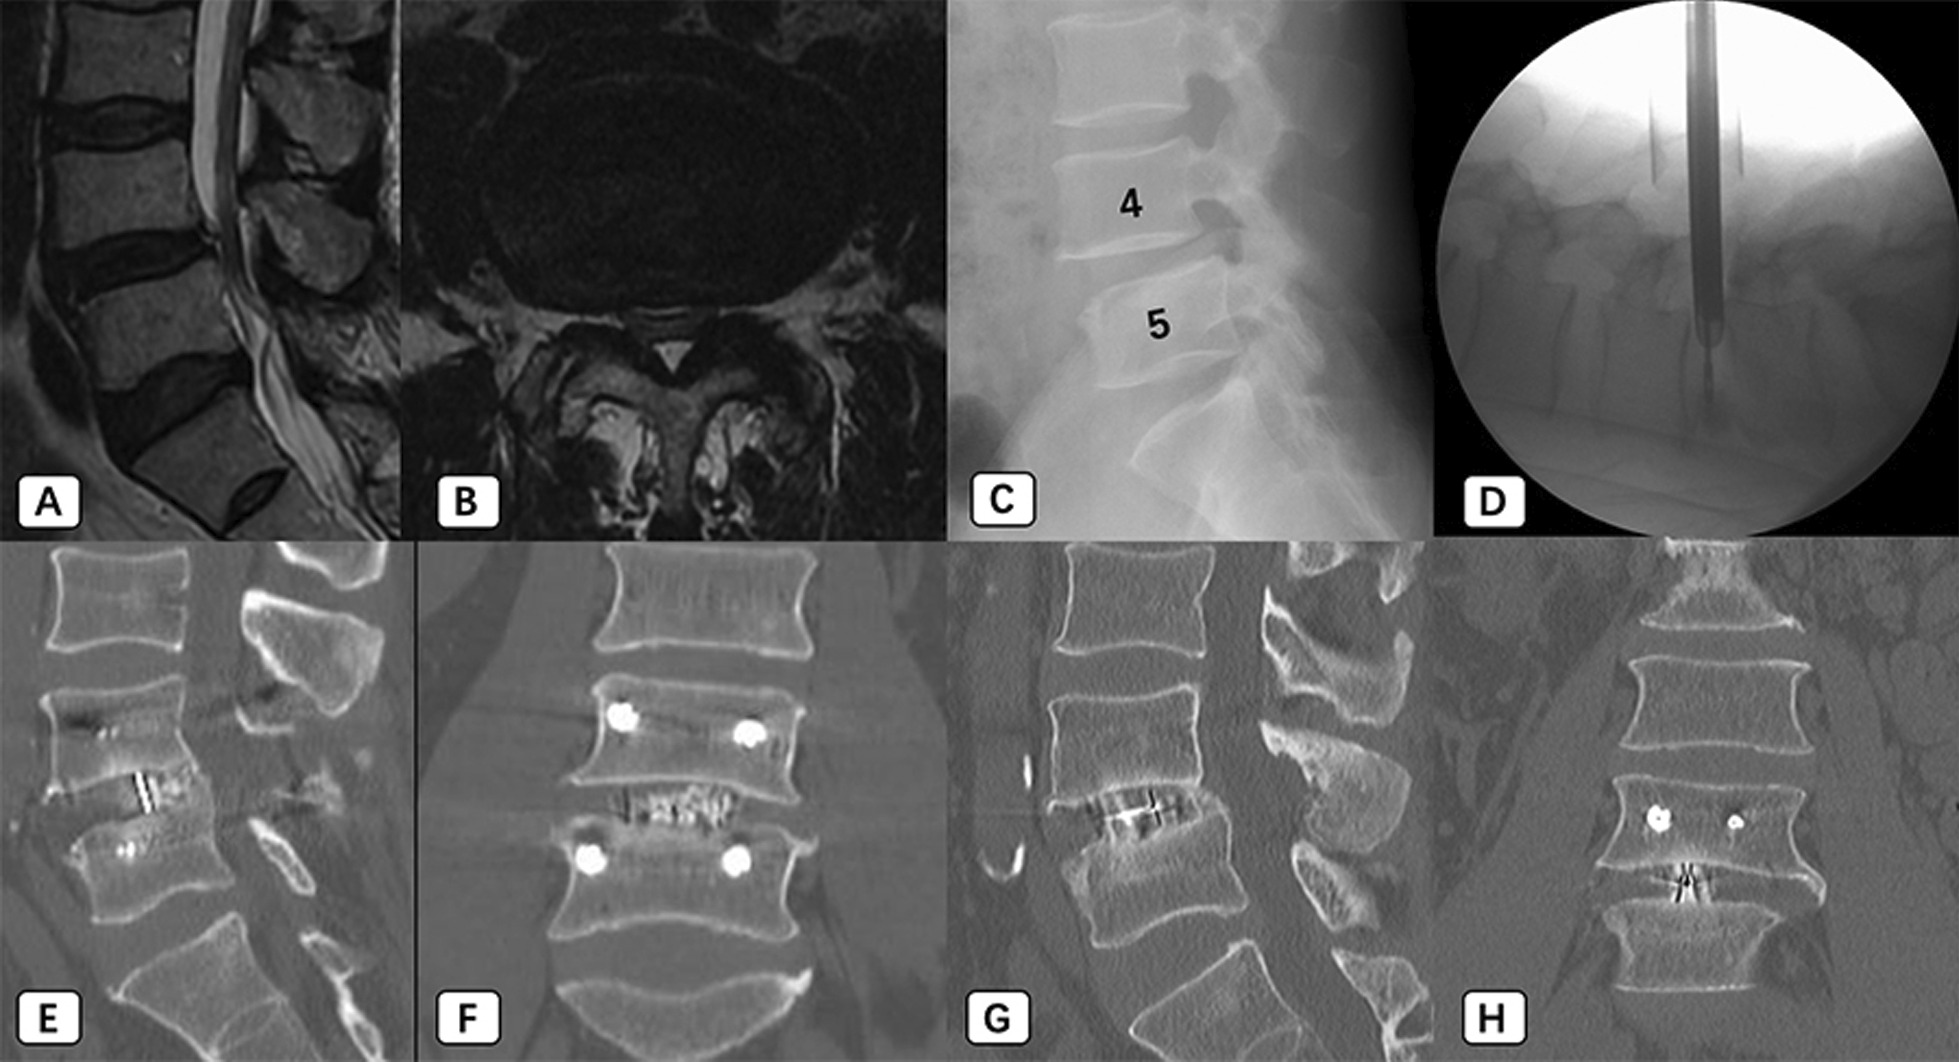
An age 50 male patient underwent endoscope-assisted minimally invasive transforaminal lumbar interbody fusion with fluoroscopy-guided technique at L4/5 level. A, B MR images show central stenosis with high-intensity zone at L4/5 level. C X-ray images show lumbar spondylolisthesis. D An endoscope was introduced to perform discectomy and endplate preparation. E–H, Bony fusion shows in sagittal and coronal computed tomography images at 6-month (E and F) and 20-month (G and H) postoperatively
